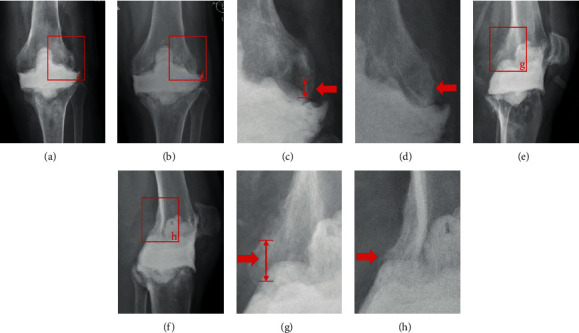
(a, e) X-ray films immediately after static spacer insertion. (b, f) Nine weeks later, X-ray films showed bone loss. The upward displacement of the spacer relative to the original joint line is the bone loss of the femur, and the downward displacement of the spacer relative to the joint line is the bone loss of the tibia. (c) and (d) show the amount of bone loss in the distal femur on the anteroposterior X-ray films. (g) and (h) show the amount of bone loss in the distal femur on lateral X-ray films.
